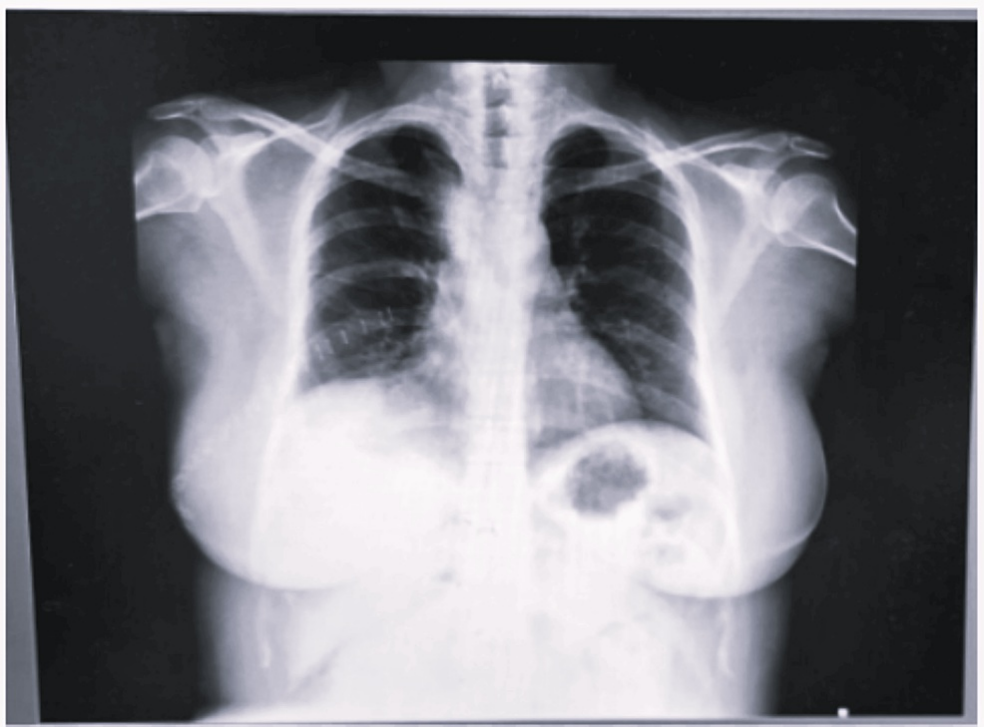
Postoperative chest X-ray PA view
